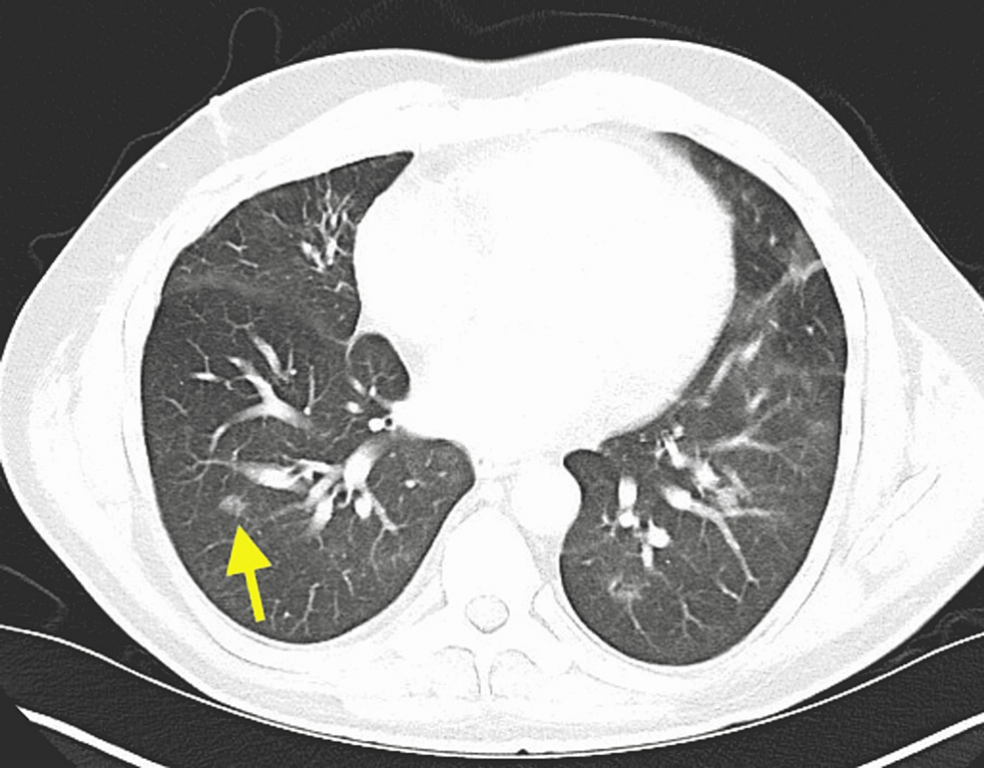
CT chest with contrast demonstrated nodular densities of the right lower lobe possibly representing septic emboli.
